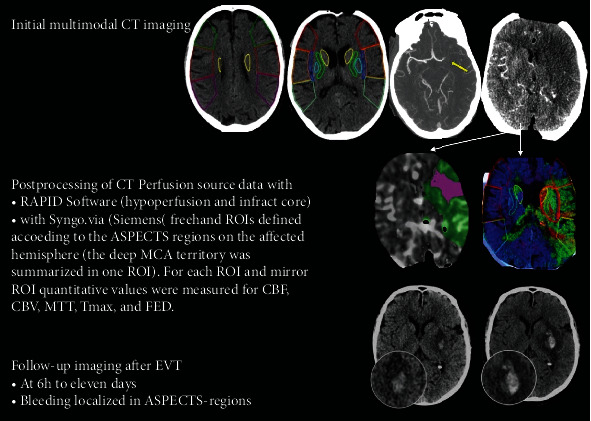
Visualization of the initial imaging, postprocessing of perfusion data, and follow-up. Abbreviations: ASPECTS, Alberta Stroke Program Early CT Score; CBF, cerebral blood flow; CBV, cerebral blood volume; EVT, endovascular treatment; FED, flow extraction product; MCA, middle cerebral artery; MTT, mean transit time; ROI, region of interest; and Tmax, maximum enhancement time.
